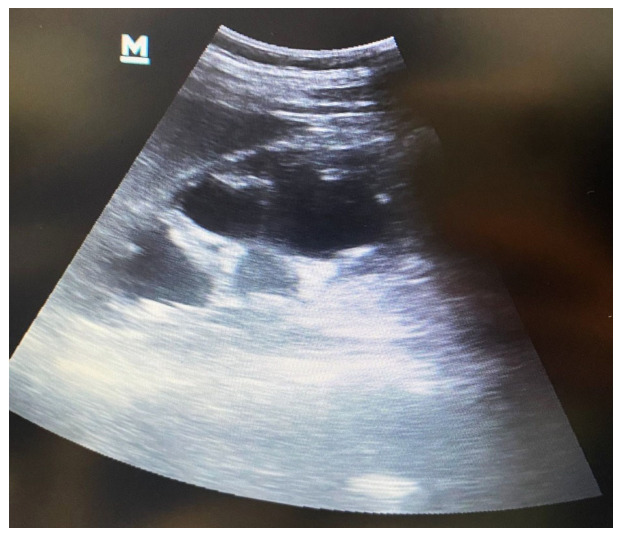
Abdominal ultrasound showed grade 4 right hydronephrosis.
